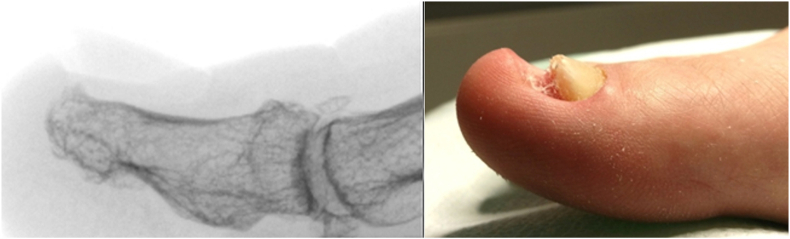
Lateral X-ray image of a bone spur of a distal phalanx with a nail growth problem.
